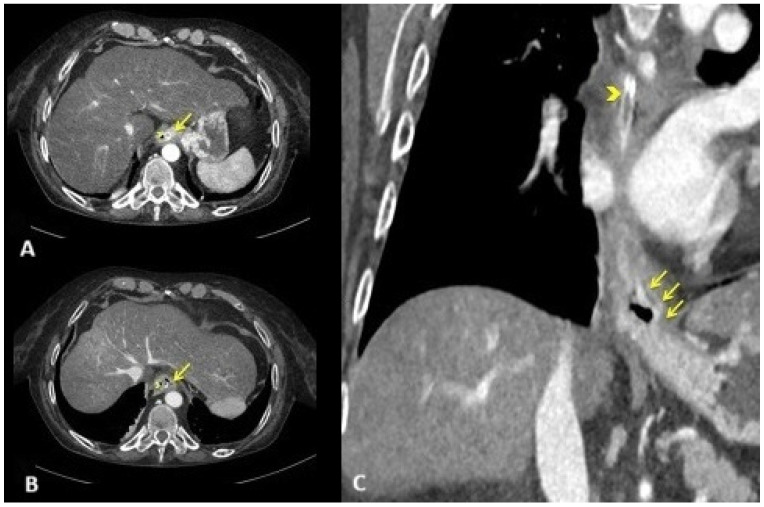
Emergent computed tomography angiography. Axial arterial (A) CT scan at the level of the gastro-esophageal junction and venous (B) CT scan at the level of lower thoracic esophagus showing circumferential thickening of the esophageal wall. Note the mucosal enhancement ((A); yellow arrow) with wall hypoattenuation, producing the target sign ((B); yellow arrow). Coronal venous CT reconstruction showing extension of esophagitis predominantly to the distal third of the esophagus, including the gastro-esophageal junction ((C); yellow arrows). Nasogastric tube ((A–C); yellow arrowhead).
